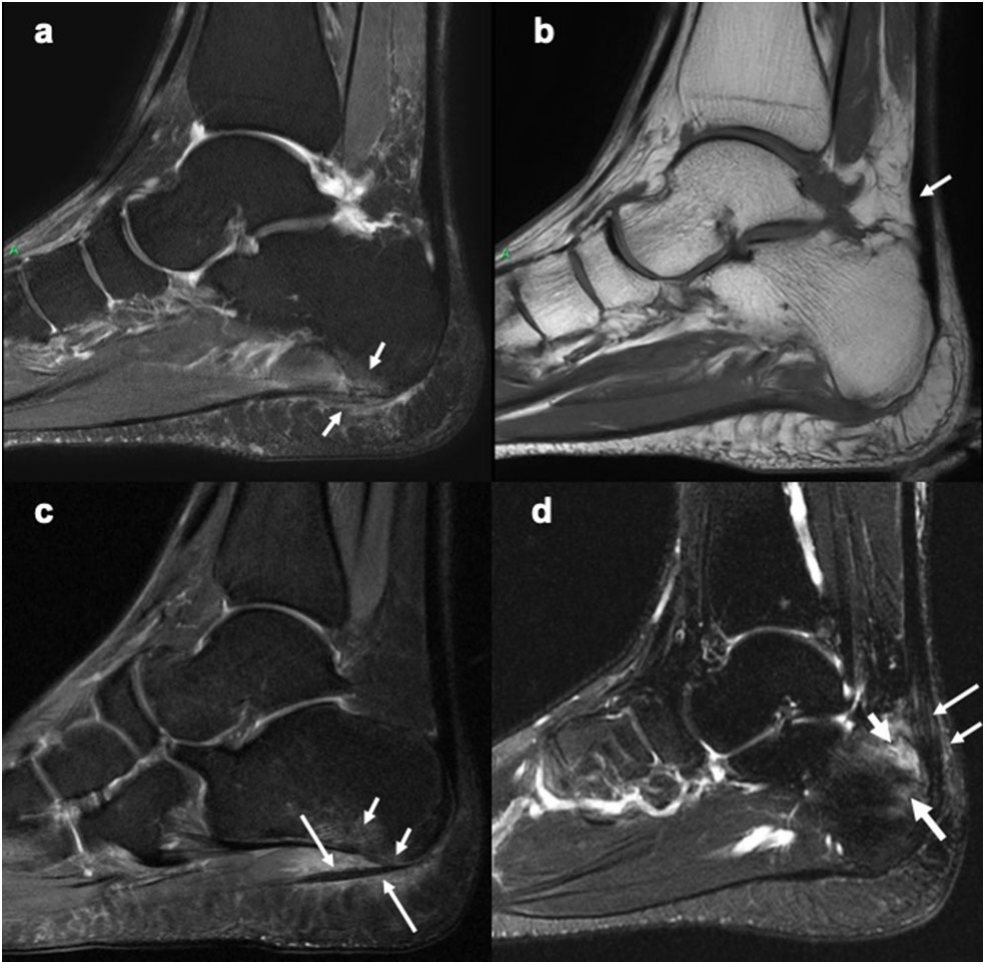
Sagittal MR images of the heel region depicting enthesitis at Achilles tendon and plantar fascia attachments. (a) STIR image showing bone marrow edema at the plantar fascia insertion to the calcaneum, intrafascia high signal intensity and perifascia high signal intensity. (b) Corresponding T1-weighted image showing probable mild thickening of a part of the Achilles tendon (arrow). (c) STIR image showing bone marrow edema (short arrows) close to the plantar fascia insertion to calcaneum and severe perifascia high signal intensity (long arrows). (d) STIR image showing bone marrow edema (long thick arrow) at the Achilles tendon insertion to calcaneum, intratendonous (long thin arrow) and, peritendonous (short thin arrow) high signal intensity, as well as retrocalcaneal bursitis (short thick arrow). (a,b) Courtesy of Professor Iris Eshed, Sackler School of Medicine, Tel Aviv University, Tel Aviv, Israel. (c,d) have been taken from the OMERACT MRI heel enthesitis exercises (27, 80).
